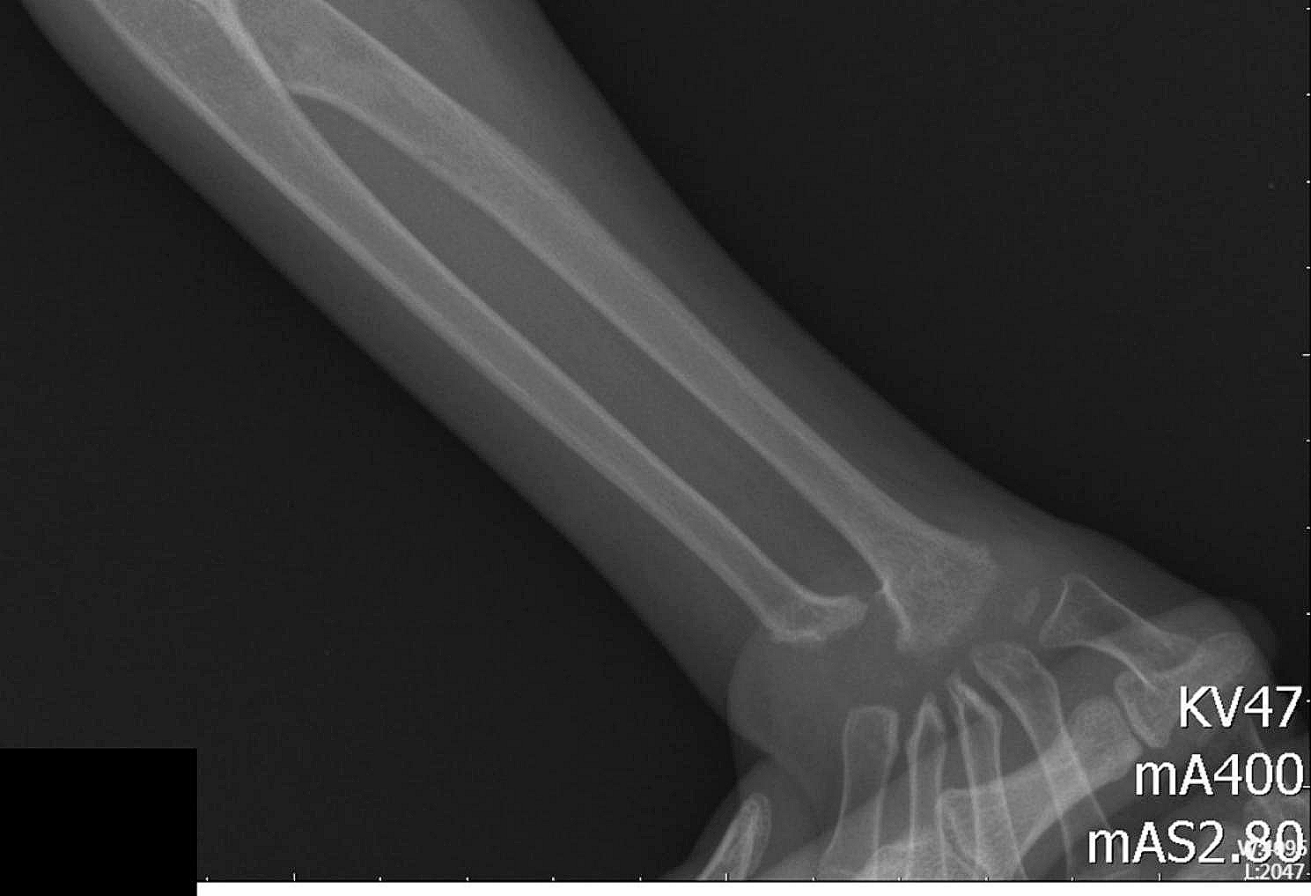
X-ray of right hand of the patient at 3 years of age showed swelling of the soft tissue of elbow joint. Deformities of the proximal ends of the metacarpals and distal ulna and radius were noted
